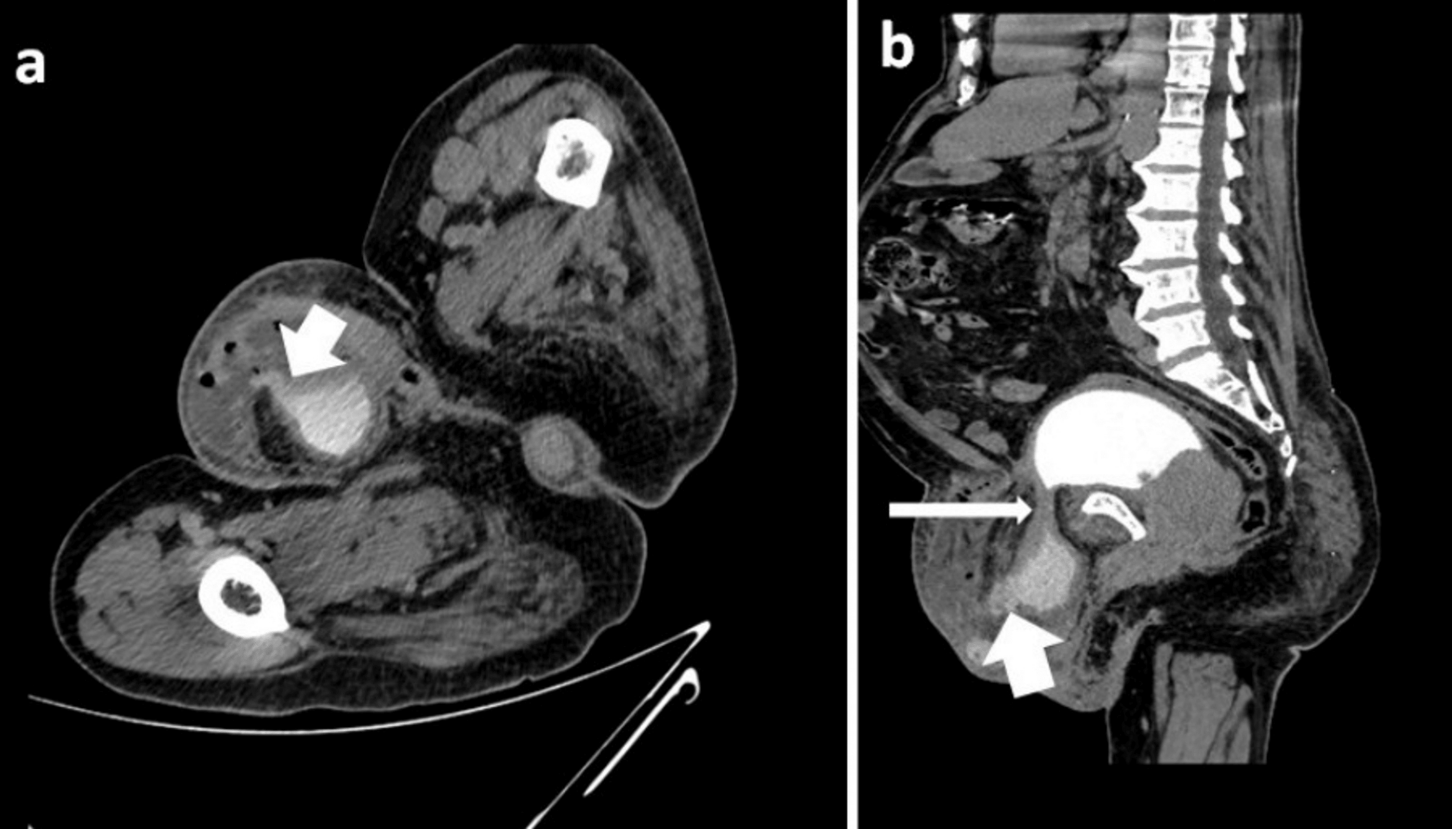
CECT abdomen and pelvis delayed phase: (a) axial section shows extravasation of contrast from the rent in the wall of herniated urinary bladder (short white arrow); (b) sagittal section shows herniation of urinary bladder into the left inguinal canal (long white arrow) and extravasation of contrast from the rent in the wall of herniated urinary bladder (short white arrow) CECT, contrast-enhanced CT
